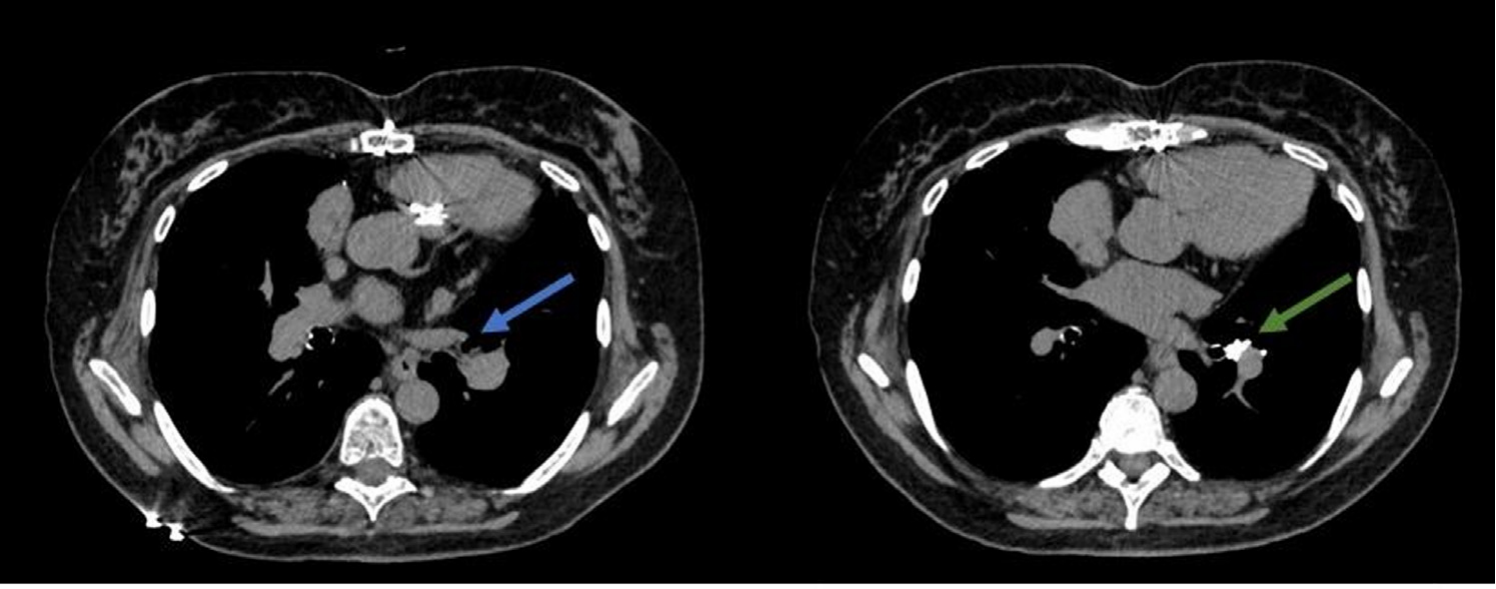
Computed Tomography Angiography of the Chest Imaging demonstrates significant enlargement of the central pulmonary arteries, consistent with chronic pulmonary valve disease. Additionally, a calcified subocclusive chronic thrombus is identified in the left lower lobe pulmonary artery. This finding, indicated by the blue and green arrows, highlights the location of the thrombus at the origin of the segmental arterial branches of the basilar segments of the left lower lobe.
